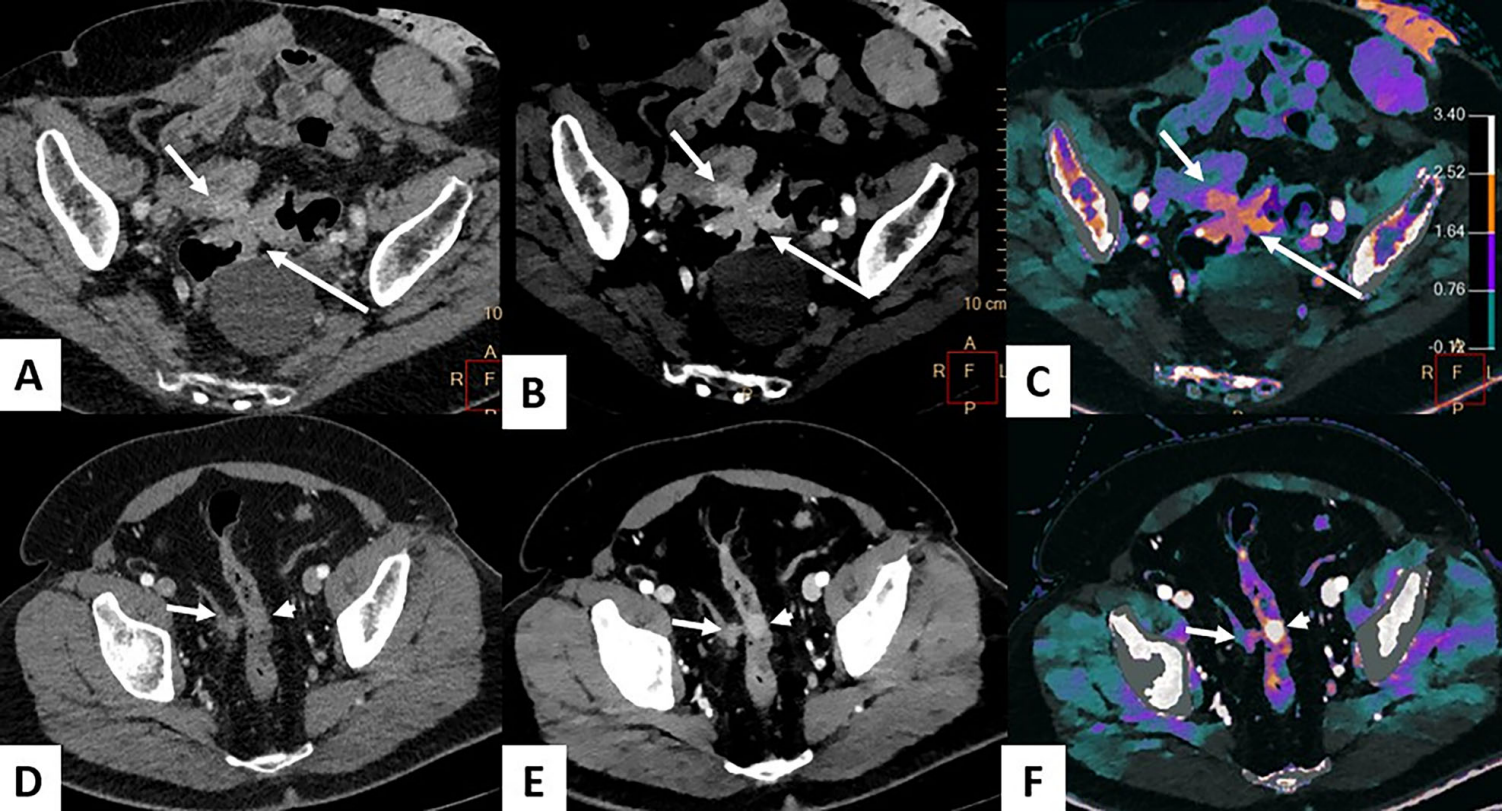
65-year-old female with synchronous PM from a sigmoid primary tumor. DLCT (Iqon, Philips) in axial section at VP, performed after NAC. A The conventional CT image shows an irregular circumferential parietal thickening of the primary sigmoid lesion (long arrow), while the nodule in contact with the small-bowel serosa is difficult to distinguish (short arrow). B The 40 keV VMI image provides better delineation of both the nodule and the primary tumor. C The iodine map image confirms significant iodine uptake in both the primary tumor and the peritoneal nodule. D The conventional CT image also reveals a 13 mm nodule in the right iliac fossa, located against the parietal peritoneum (arrow), while the nodule adjacent to the serosa of the distal sigmoid is poorly visualized (arrowhead). E The 40 keV VMI image enhances the contrast between the peritoneal nodule (arrow), the sigmoid serosal nodule (arrowhead), and the adjacent structures. F The iodine map image shows significant iodine uptake in the parietal peritoneal nodule (short arrow), and especially in the nodule of the sigmoid serosa (arrowhead). CRS, cytoreductive surgery; CT, computed tomography; DLCT, dual-layer computed tomography; NAC, neoadjuvant chemotherapy; PM, peritoneal metastases
